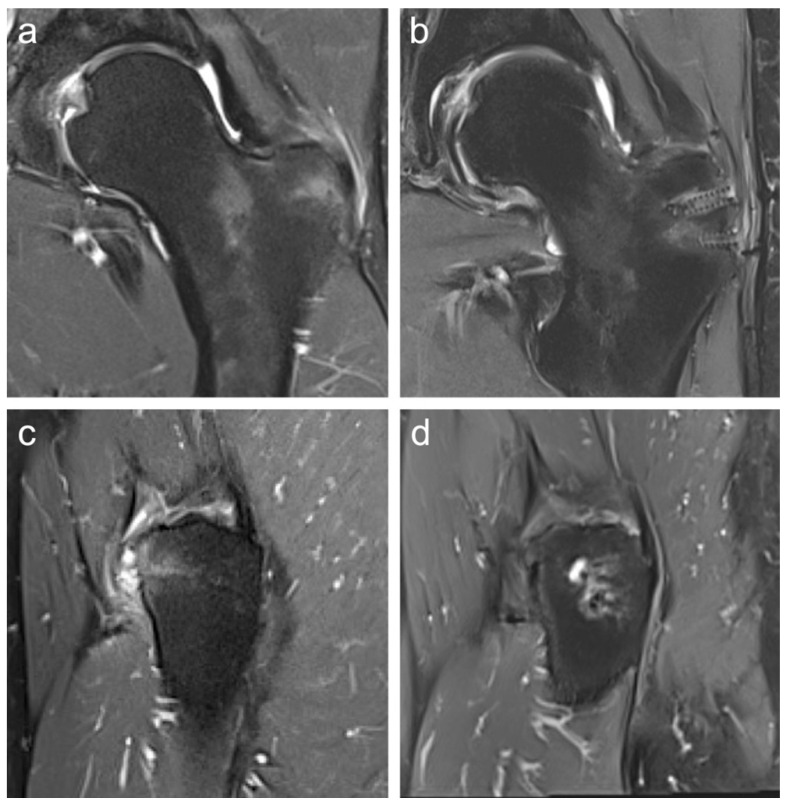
Preoperative coronal (a) and sagittal (c) 3-Tesla MRI showing a partial rupture of the GMin and GMed tendon insertions at the greater trochanter. Postoperative coronal (b) and sagittal (d) 3-Tesla MRI confirm intact suture anchor placement, complete tendon reattachment, and absence of re-rupture, seroma, or recurrent bursitis.
